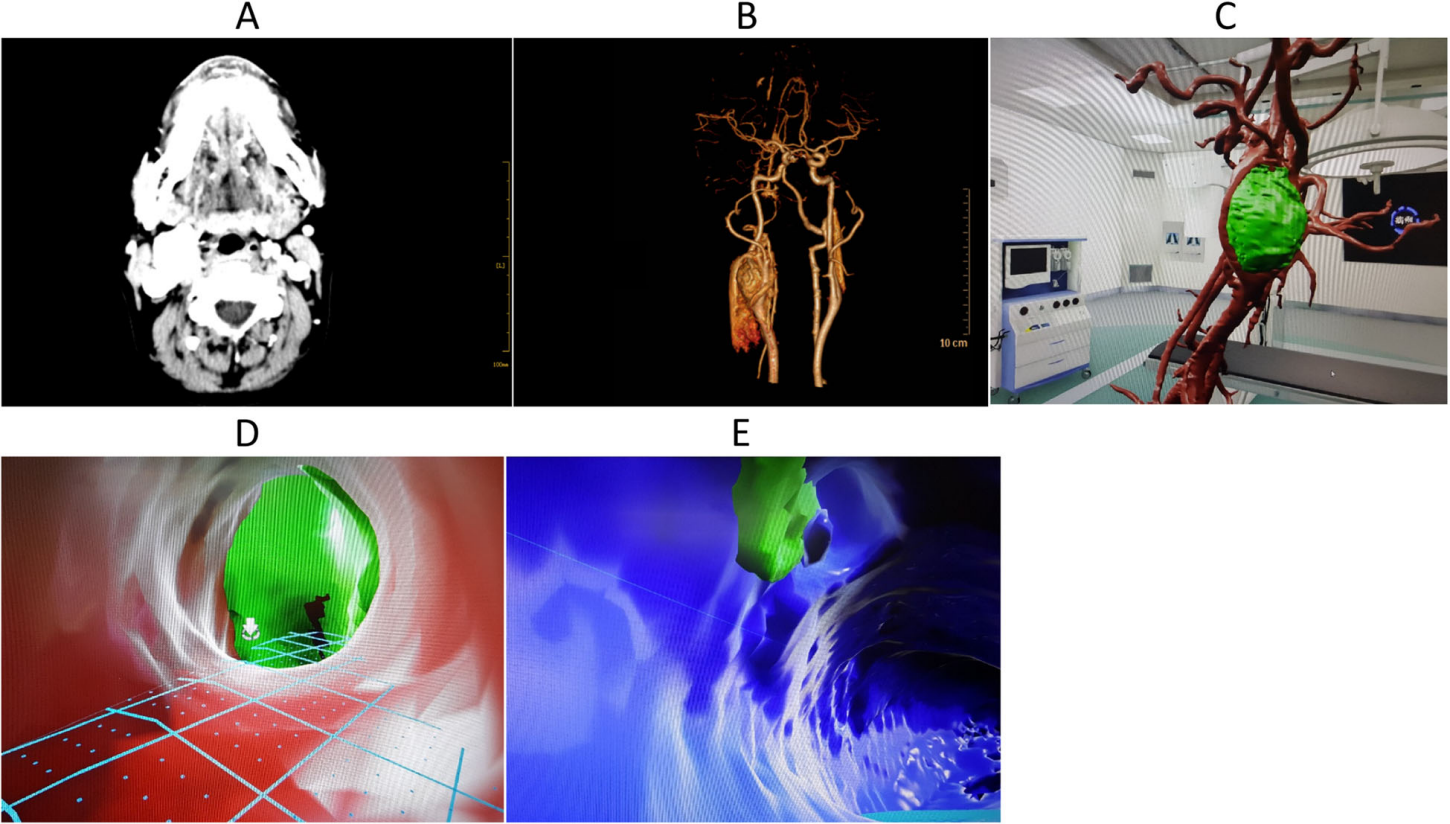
a CT shows the relationship between tumor and adjacent tissue; b CTA shows the relationship between tumor and blood vessel; c VR model after removal of the venous system; d Intravascular peep from the internal arteries of the carotid body tumor; e Intravascular peep from the vein of the carotid body tumor
